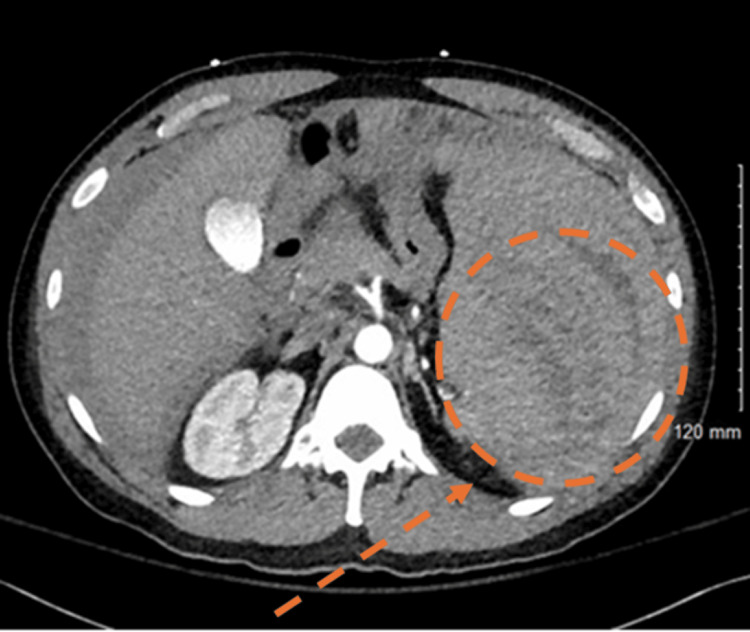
Transverse CT scan of the abdomen Highlights a splenic rupture, with evidence of disrupted splenic parenchyma and associated changes suggestive of intra-abdominal hemorrhage. The affected area is circled for clarity.
